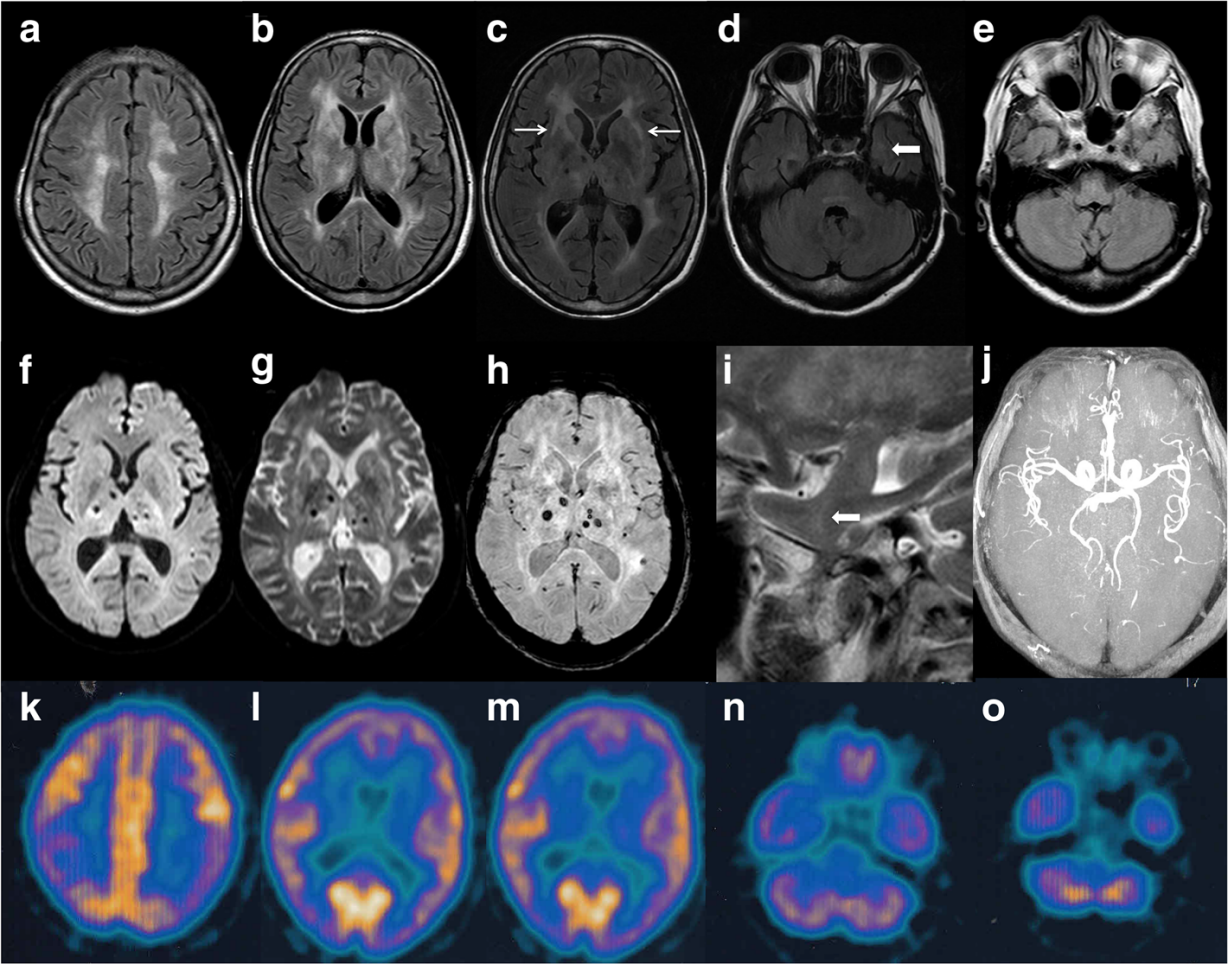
The patient’s cranial imaging findings. a-j Magnetic resonance imaging showed abnormal signals in the bilateral frontoparietal subcortical area, periventricular region of the lateral ventricle, basal ganglia, and dorsal thalamus. The bilateral external capsules (c) and left temporal pole d and i were affected as indicated by *arrows*. h, SWI revealed multiple hemosiderin depositions or minor hemorrhage lesions in the bilateral basal ganglia, dorsal thalamus, and right parietal lobe. j, MRA indicated reduced distal branches of the cerebral arteries. k-o, 18Fluoro-deoxy-glucose-PET/CT showed reduced glucose metabolism in the bilateral lobes, basal ganglia, and thalamus
